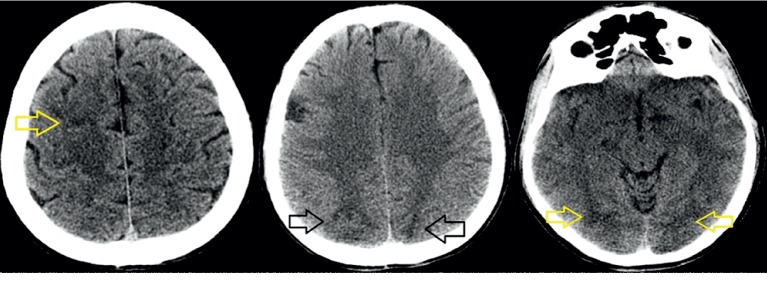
CT scan images: bilateral subcortical hypodensities in frontal, occipital, and parietal regions.
